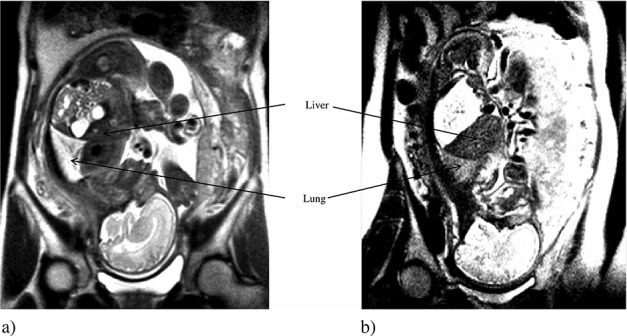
Images of lung and liver on T2-weighted magnetic resonance imaging. (a) Non-severe respiratory disorder (SRD) case at the 32^nd^ week of gestation, lung-to-liver signal intensity ratio (LLSIR) = 2.241. (b) SRD case at the 32^nd^ week of gestation, LLSIR = 1.418
